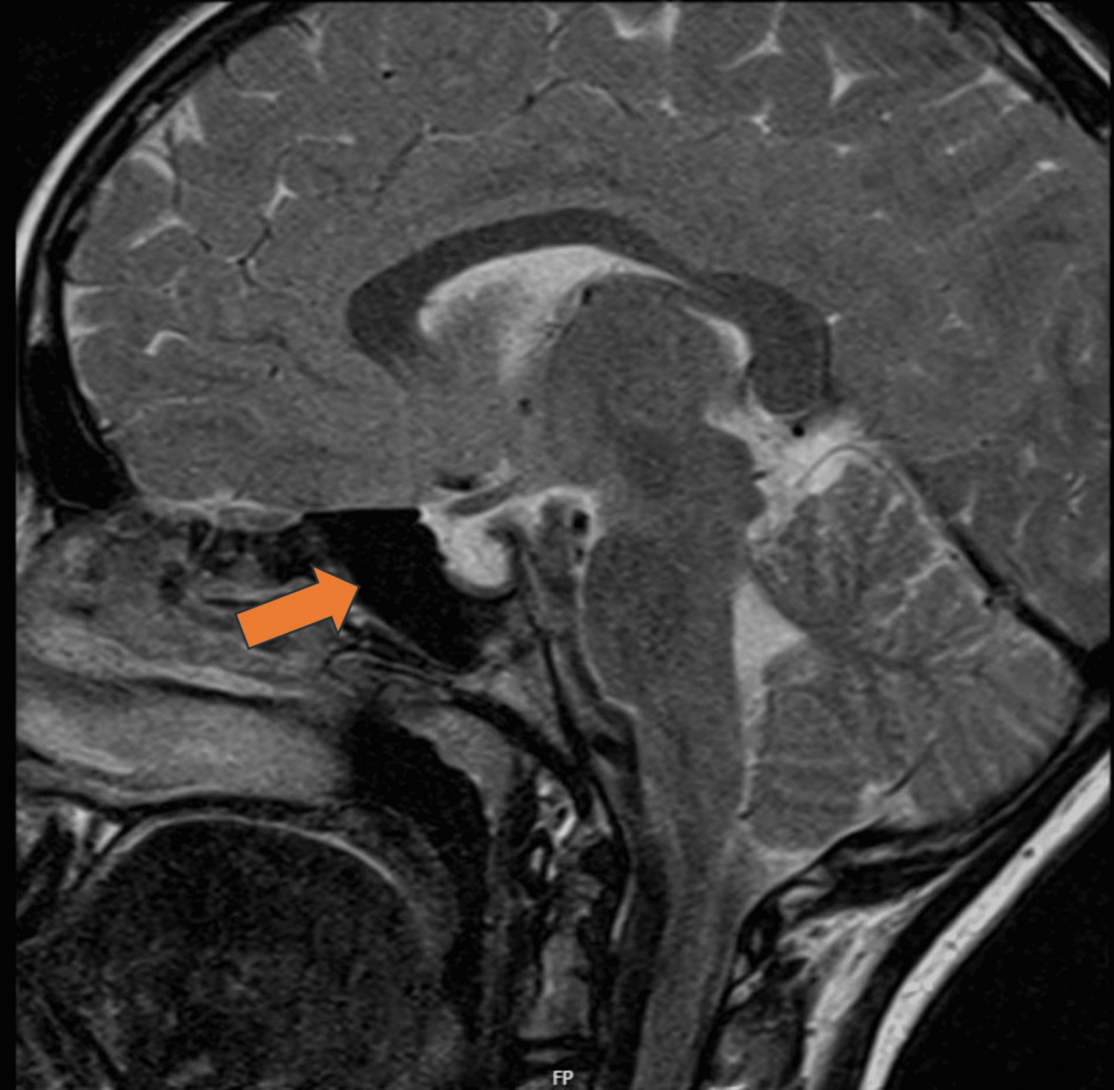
Empty sella turca on MRI
